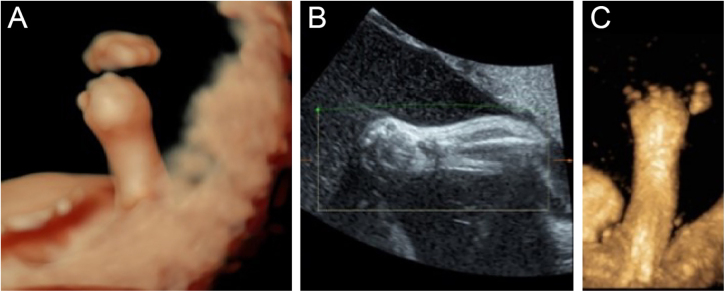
(A) 3-D ultrasound: clenched hands and skin oedema. (B) 2-D ultrasound: a shortening of phalanges and metacarpals. (C) 3-D ultrasound: the image of the upper limb of the fetus
